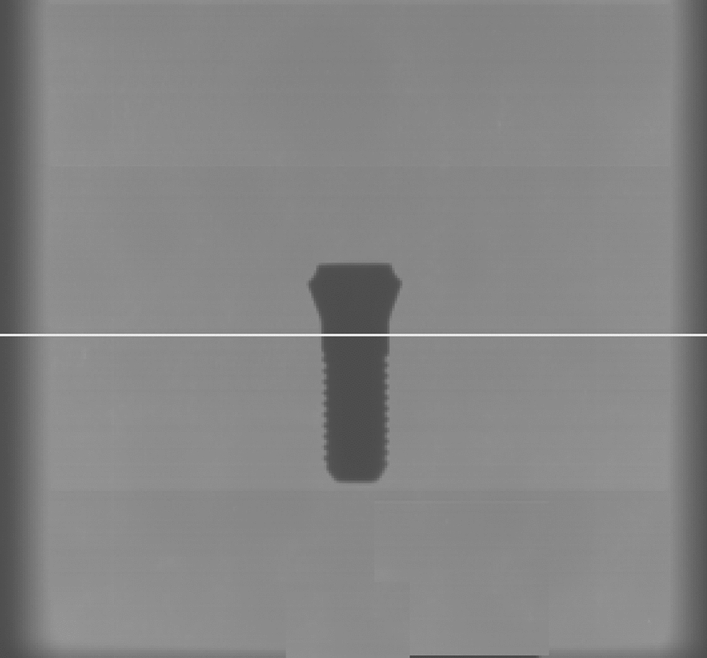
Exemplary 2D projection radiograph from the 3D Accuitomo with the Y-TZP-implant positioned in the center of the FOV. The white line represents the line along which the gray value profile was measured and evaluated, so that in eventually only the values within the implant image were used for computing transmission.
